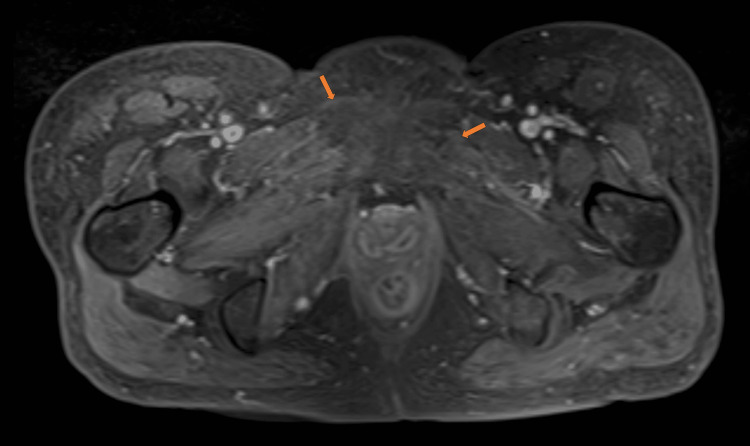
Contrast-enhanced MRI axial image after the first course of hyperbaric oxygen therapy Resolution of the mass in the left obturator externus muscle and right pectineus muscle (orange arrows). MRI: magnetic resonance imaging
